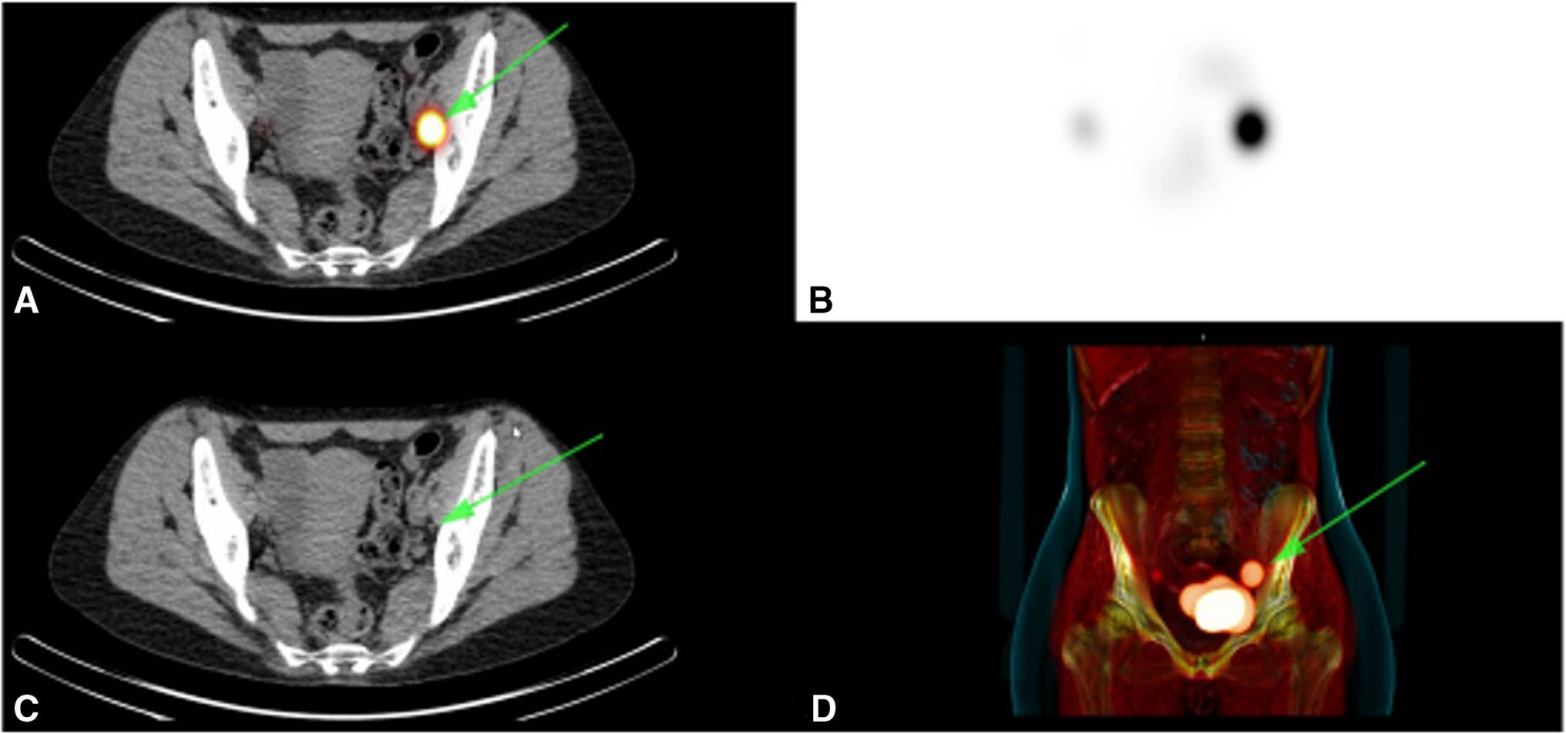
Preoperative SPECT/CT SN imaging. A: fusion of low-dose CT and SPECT image with localization of SN (green arrow). B SPECT imaging (C) Low dose CT with localization of SN (green arrow). D A 3D volume rendering of SPECT/CT image. In this patient, an intense SN was seen on the left obturator region marked with by the green arrow, on the right a faint SN was seen on SPECT/CT in the right obturator region, as better shown in the 3D volume rendering.. Abbreviations: CT = computed tomography; SPECT = Single photon emission computed tomography; SN = Sentinel node
